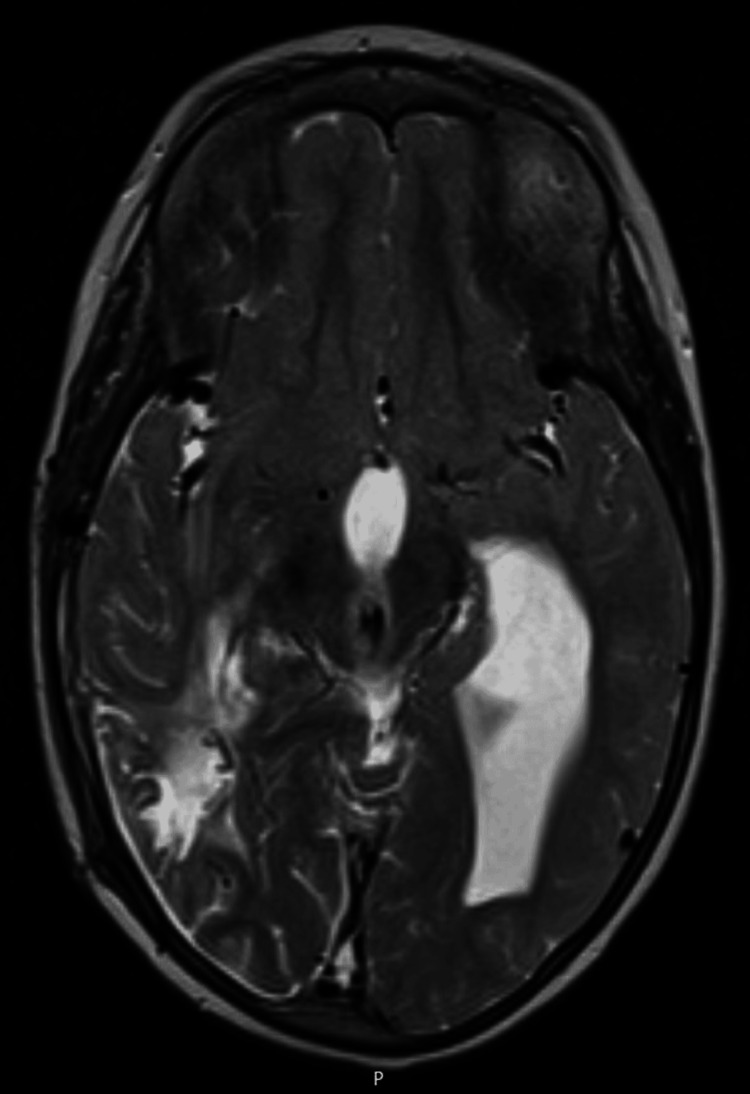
Axial T2-weighted post-surgical MRI
